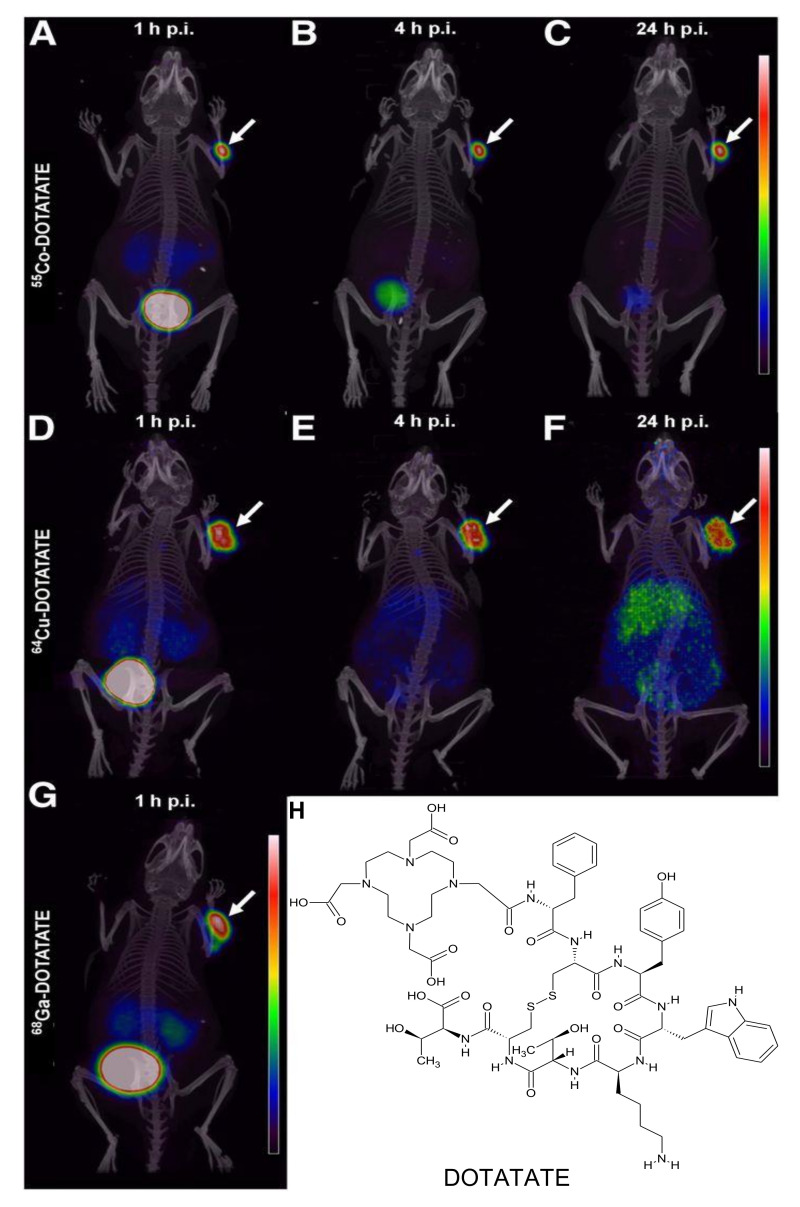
PET/CT scans comparing [^55^Co]Co-DOTATATE (A–C), [^64^Cu]Cu-DOTATATE (D–F), and [^68^Ga]Ga-DOTATATE (G) in AR42J tumors. Imaging showed similar tumor uptake using all 3 agents at 1 h PI. Imaging at later timepoint images showed near-complete non-target clearance using [^55^Co]Co-DOTATATE while [^64^Cu]Cu-DOTATATE showed non-specific liver uptake [15].
